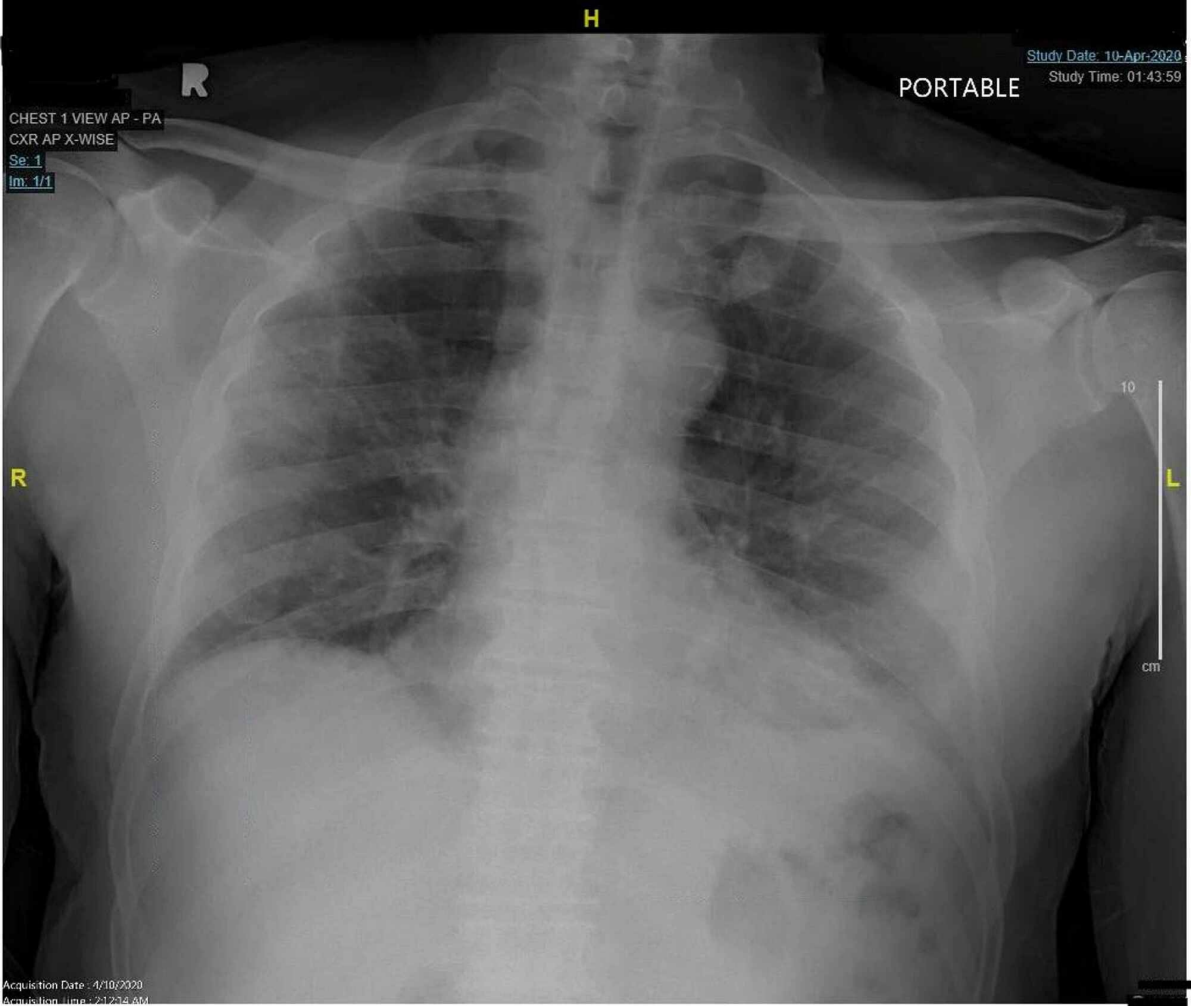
Chest x-ray on admission
